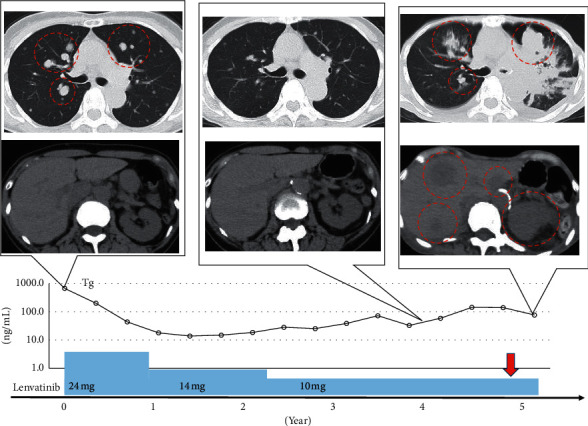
Clinical course of Case 2. The upper image is a chest computed tomography (CT) image. The patient was classified as partial response after treatment with lenvatinib, but then was reclassified as progressive disease after anaplastic transformation and became left atelectasis. The lower image is a CT image of the upper abdomen, and multiple liver metastases and metastases to the left adrenal gland and kidney appeared after anaplastic transformation. The graph below the image shows the transition of thyroglobulin, the timing of lenvatinib treatment and the dose. The red dotted circle indicates the lesion. The red arrow indicates the time of anaplastic transformation.
